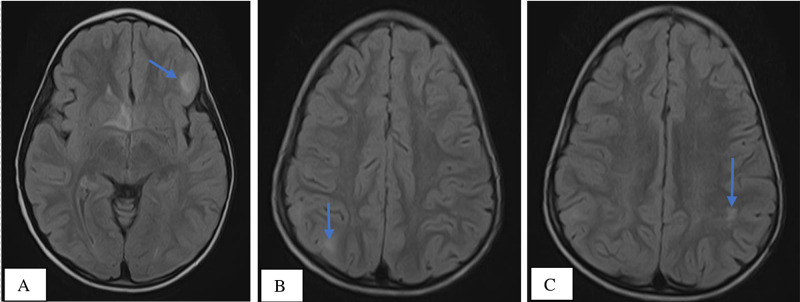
MRI, Axial FLAIR images showing well-defined ovoid hyperintense lesion measuring (1.3 x 0.7) cm in the subcortical region (grey-white matter interface) of the left frontal lobe (7A), a similar but smaller lesion-measuring (0.6 x 0.4) cm is seen in the subcortical region of the right parietal lobe (7B) and the left parietal lobe (7c) respectively all representing subcortical tubers (blue arrows).
